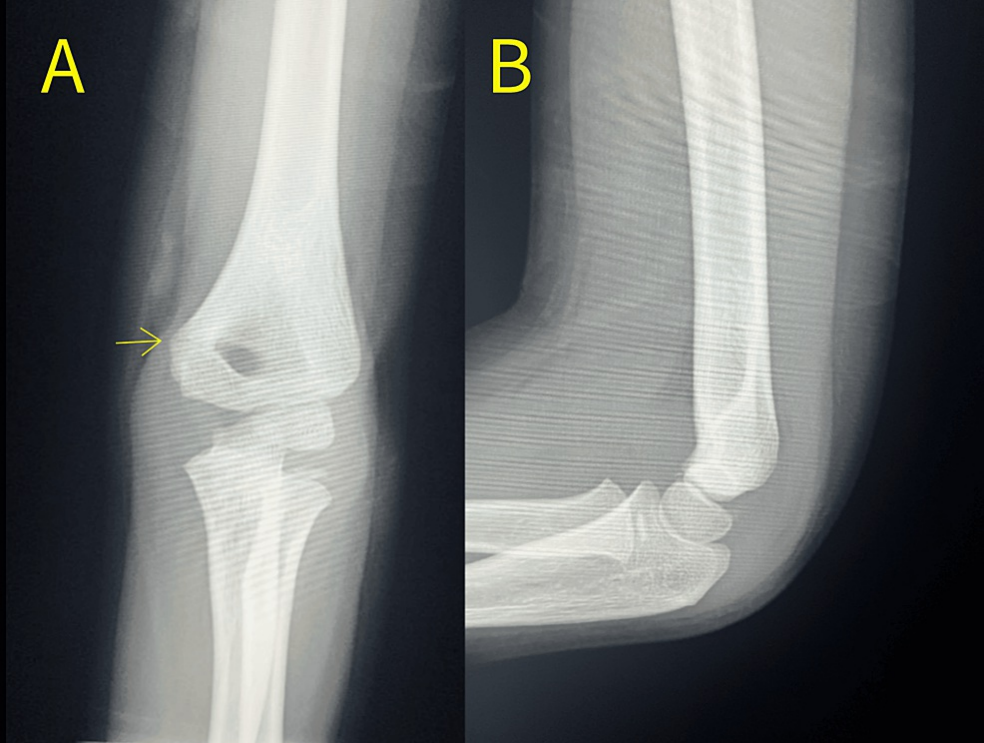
X-ray of anteroposterior (A) and lateral (B) views of the elbow joint on the day of initial injury. Anteroposterior view (A) shows a supracondylar fracture of the distal humerus without displacement.
